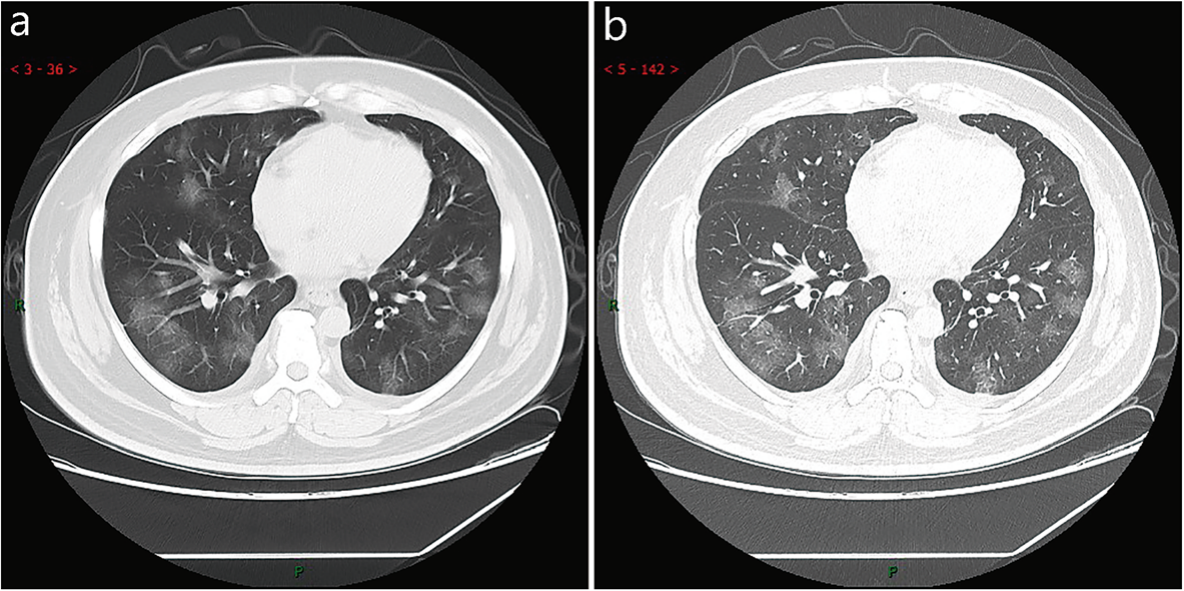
CT imaging of early stage. Male, 38 years old, fever without obvious inducement (39.3 ℃), dry cough and shortness of breath for 3 days. Laboratory test: decreased white blood cells (3.01 × 10^9^/L), decreased lymphocytes (0.81 × 10^9^/ L), increased C-reaction protein (60.8 mg/L), increased procalcitonin (0.16 ng/ml). Imaging examination: a (thin layer CT) and b (high-resolution CT) showed multiple patchy and light consolidation in both lungs and grid-like thickness of interlobular septa
